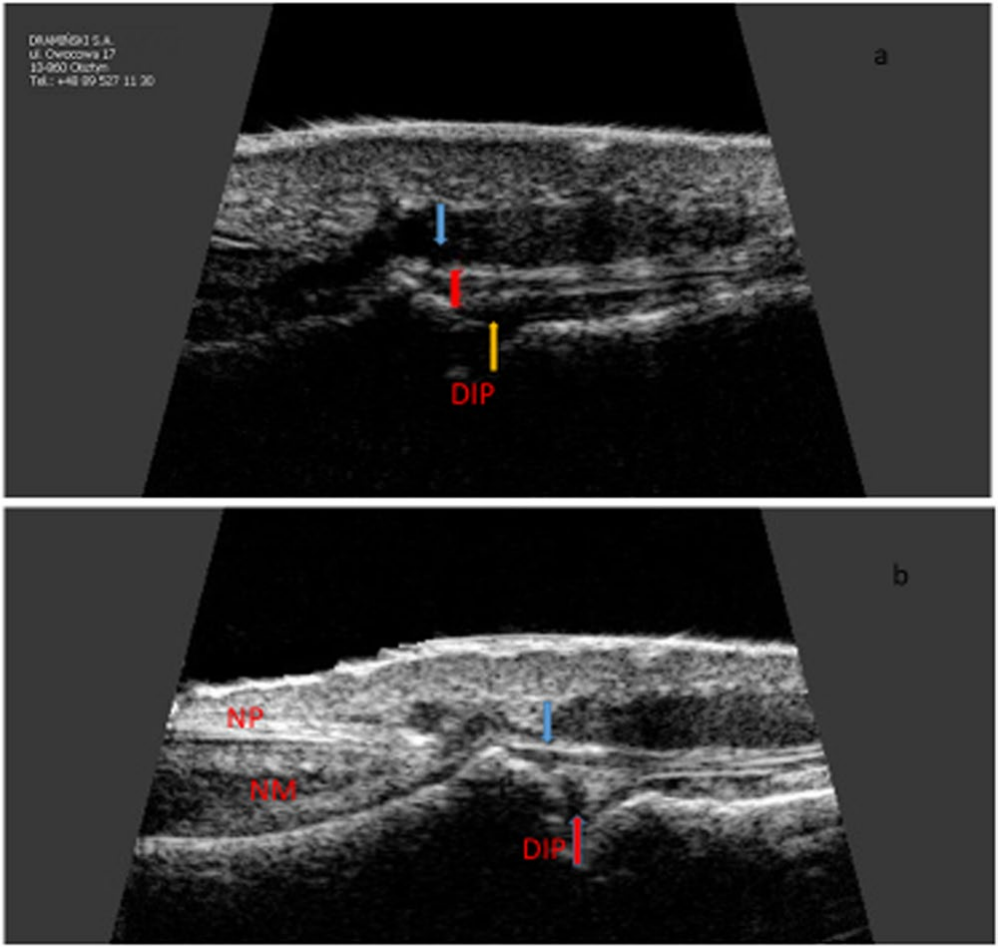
Longitudinal scan of DIP and finger extensor tendon in psoriatic arthritis. (a) Extensor tendon calcification (blue arrow), thickeness of the finger extensor tendon (red line), osteophyte (yellow arrow). (b) Bony erosion (blue arrow), loss of normal fibrillary architecture of extensor tendon. DIP: distal interphalangeal joint; NP: nail plate; NM: nail matrix. Sonographic image obtained by MK-W.
